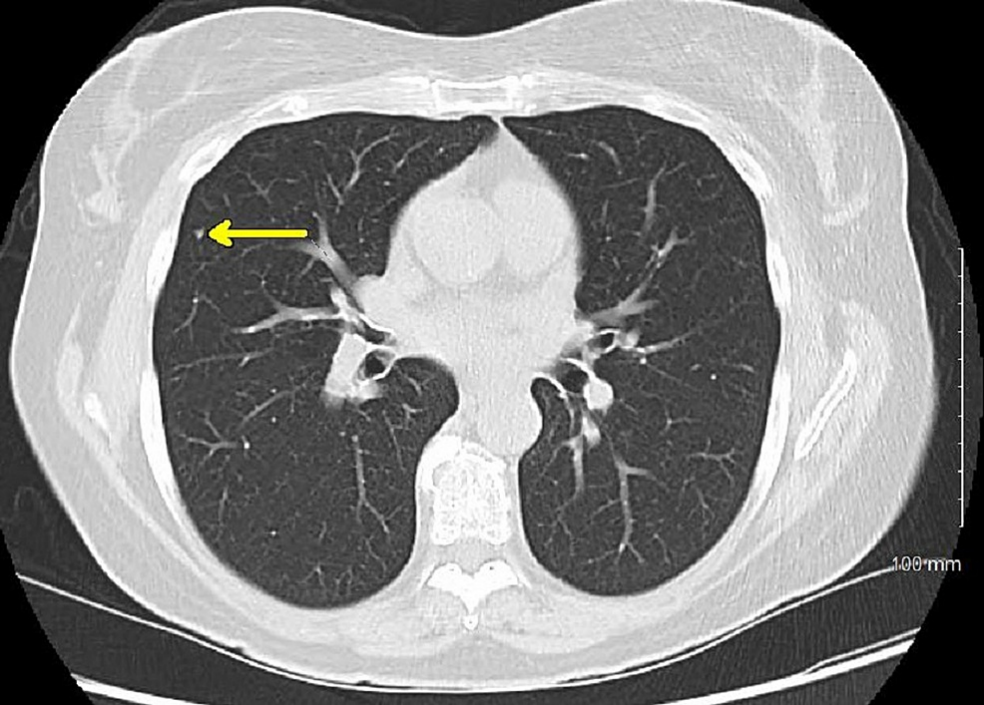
CT scan in the axial view demonstrating sub-centimeter nodule (arrow) in the right upper lobe CT: computed tomography
